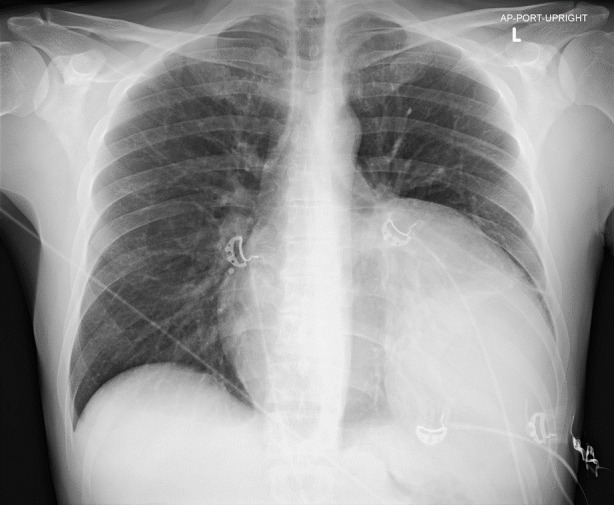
A plain film radiograph of the chest revealing cardiomegaly with a left lower lobe opacity versus mass outlined by arrows.
